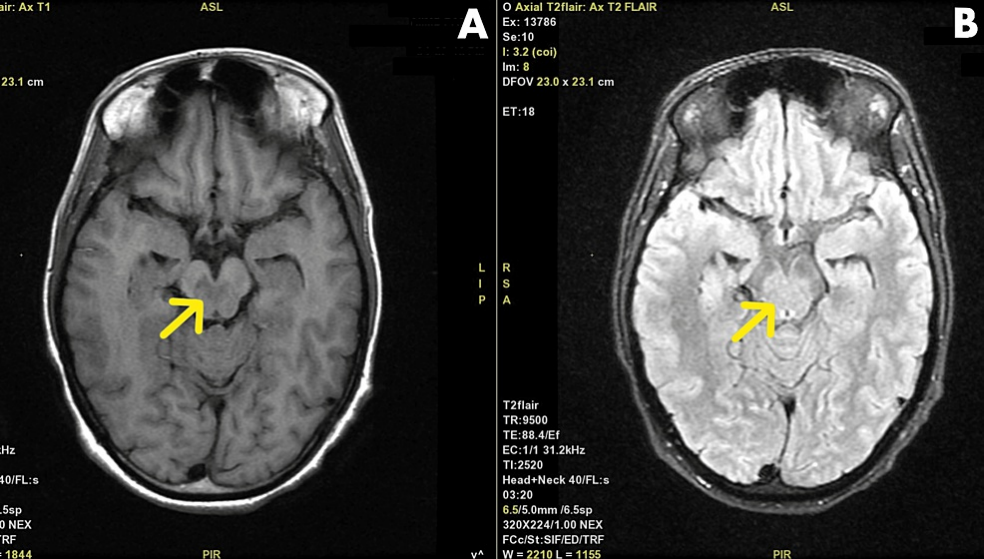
Brain MRI of the patient showing (A) an axial section of T1-weighted image showing hypointensity in bilateral tegmentum and (B) a T2-FLAIR image showing hyperintensity in the corresponding area. The arrows in both panes A and B point to the areas showing the features mentioned in the corresponding panes. MRI, magnetic resonance imaging; T2-FLAIR, T2-weighted fluid-attenuated inversion recovery
